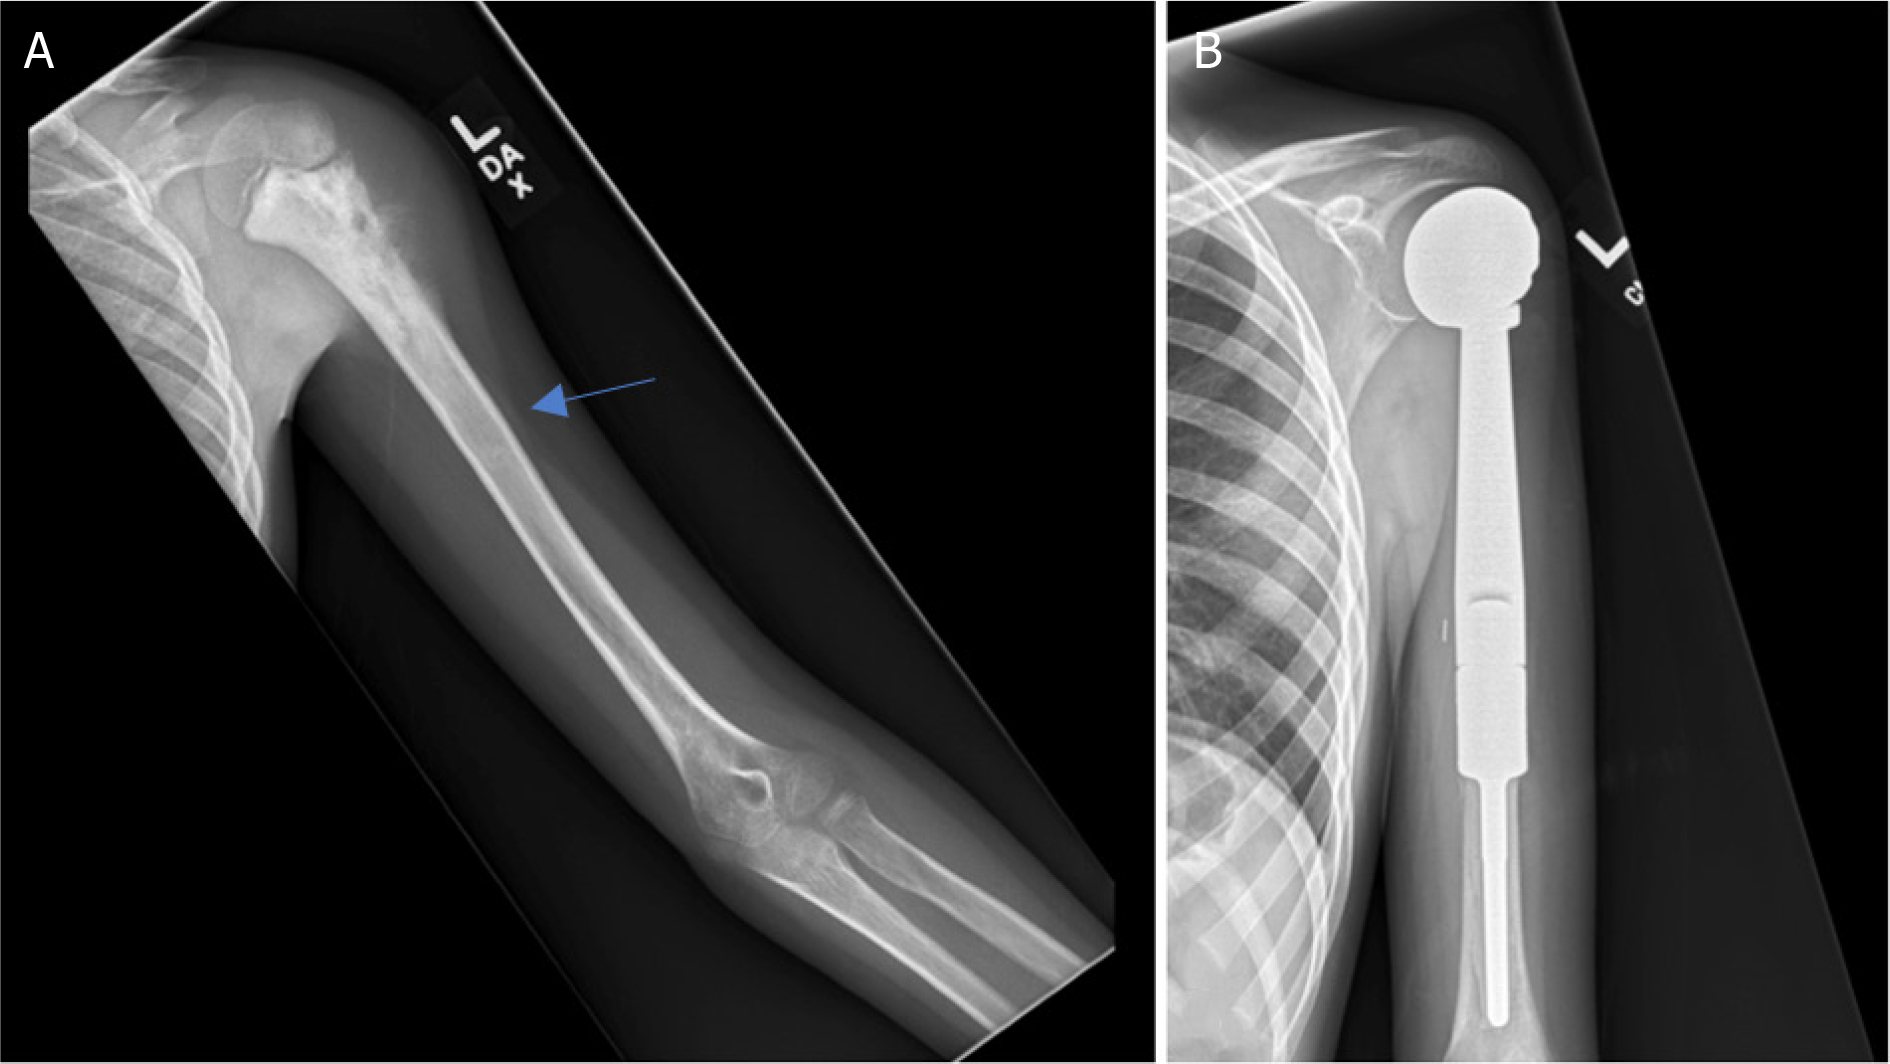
Anteroposterior radiographs of a 10-year-old male with: osteosarcoma of the proximal humerus preoperatively (A); after wide excision and proximal endoprosthesis reconstruction (B) Blue arrow notes the amount of resected bone
